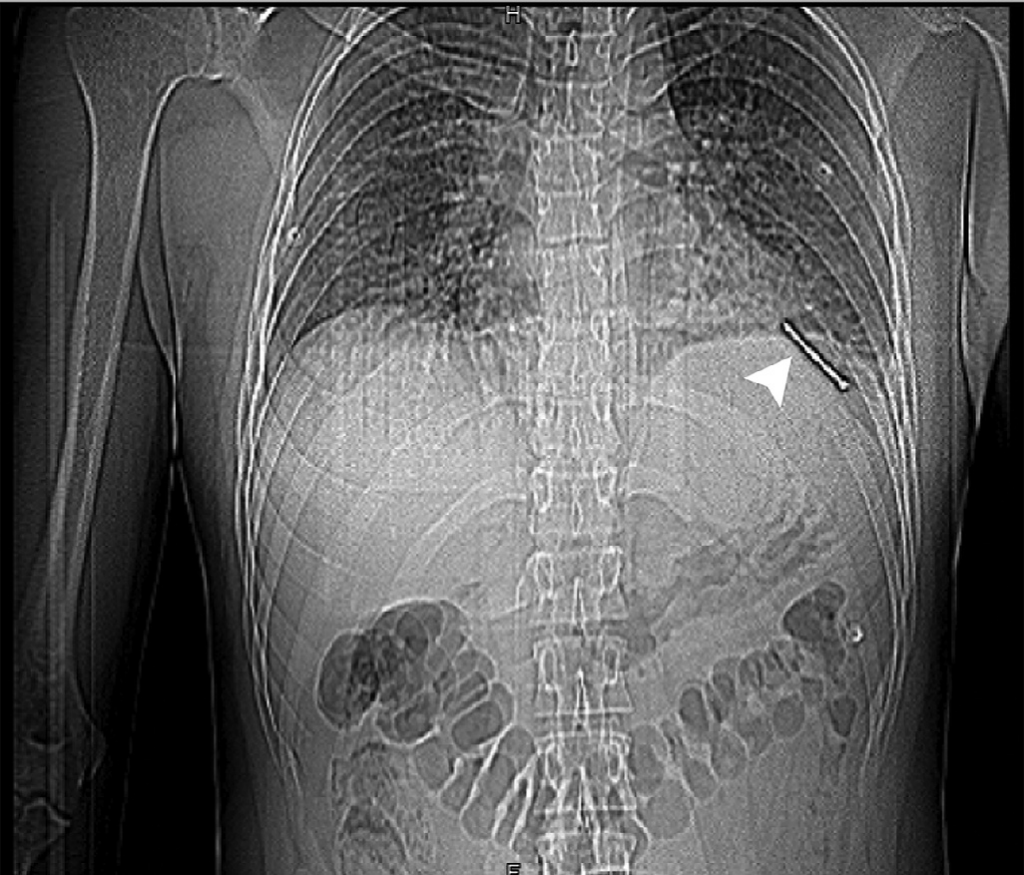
AP scout view of the chest CT showed the whole nail route in the chest (white arrowhead).
